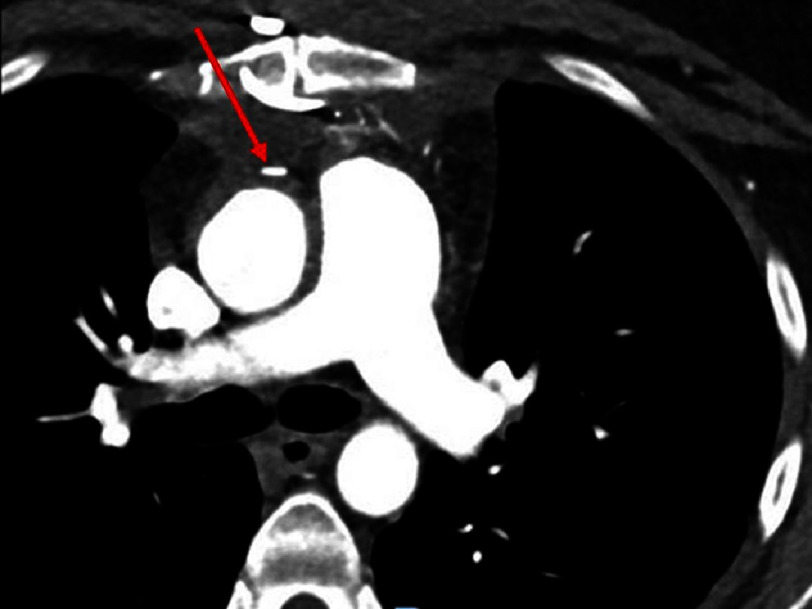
Axial image of computed tomography scan of chest (coronary CT) at the level of the pulmonary bifurcation demonstrates radiopaque marker anterior to the ascending that marks the proximal anastomosis. There is no contrast seen in the saphenous vein graft (SVG) originating from the aorta at this location that was sewn to the posterior descending artery (PDA) during coronary artery bypass grafting (CABG).
